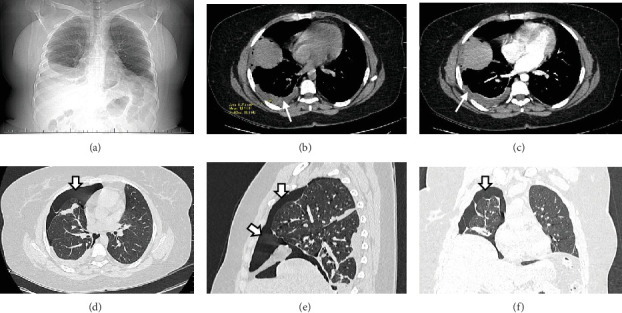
(a) Scanogram shows right-sided pleural effusion. (b) An axial chest CT scan, soft tissue window noncontrasted and (c) contrasted, showed free fluid collection in pleural space (14HU) involving the right hemithorax in keeping with right pleural effusion (long arrows). Additionally, there is a pleural-based nonenhancing hemorrhagic mass was observed within the middle lobe. (d–f) Lung windows showed peripheral air-dense region devoid of lung markings (short arrows) in the right hemithorax (pneumothorax) with underlying right lung collapse and areas pulmonary fibrotic changes.
